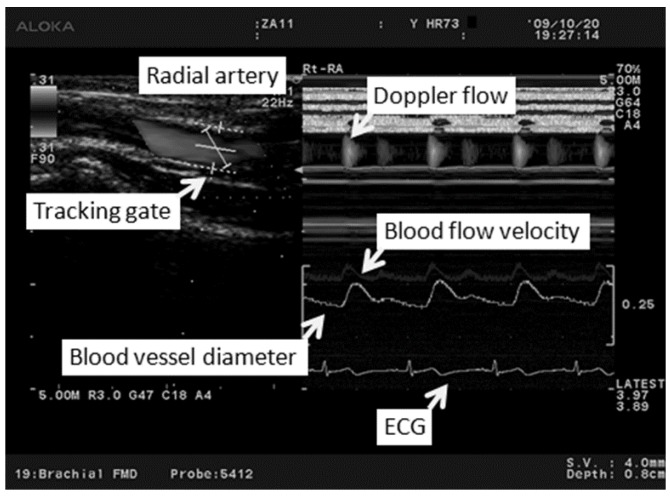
The image on the left shows the radial artery and the position of the tracking gate on the artery. The image on the right shows the changes in the vessel diameter, Doppler flow and flow velocity determined using an automated edge detection device and computer analysis software.
